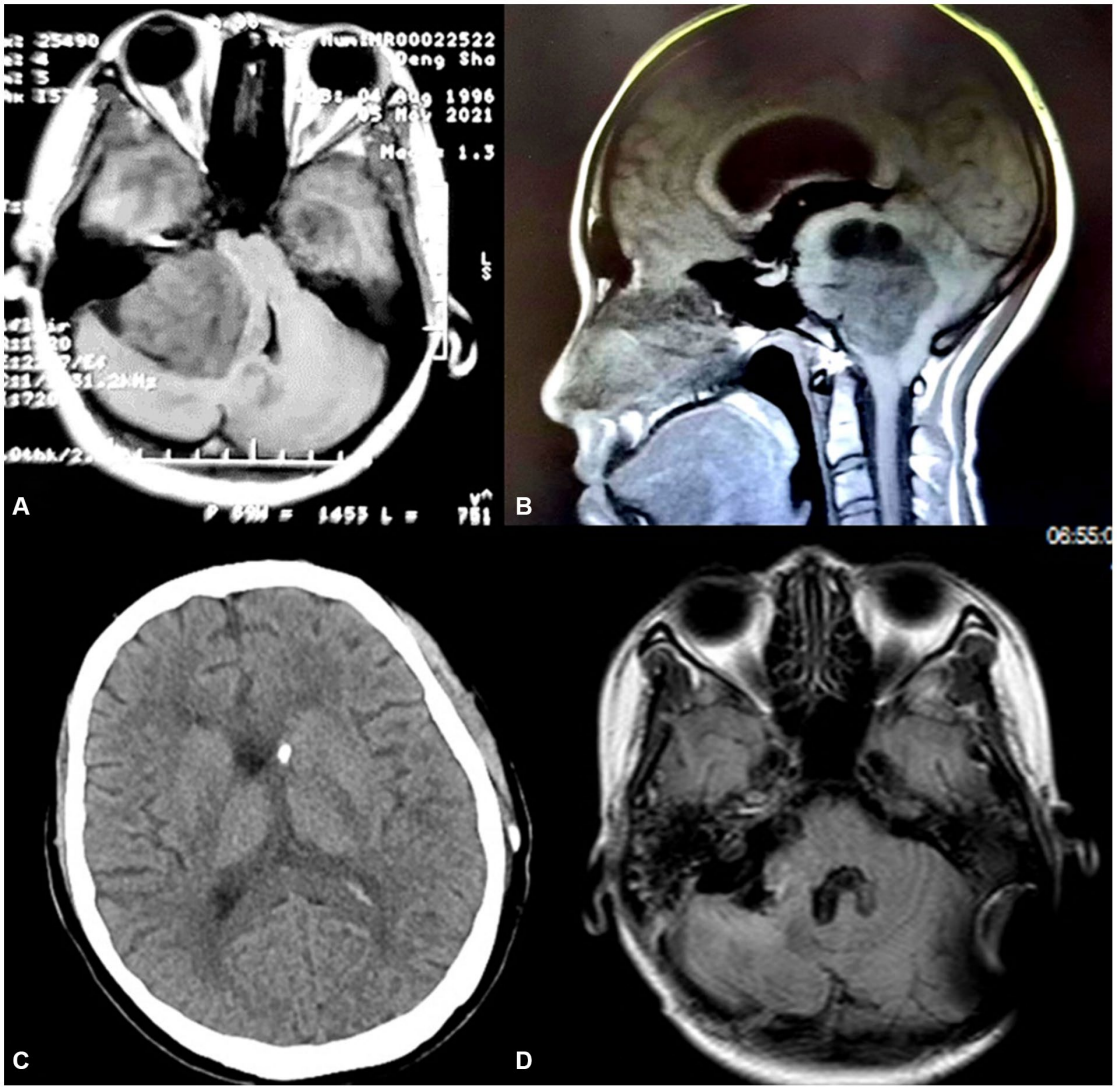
(A,B) There is a large solid mass about 6*5 cm in size in the right CPA area with ventricular and brainstem compression; (C) After the V-P shunt, CT revealed significant relief of her hydrocephalus; (D) Postoperative magnetic resonance imaging shows complete removal of the tumor.
